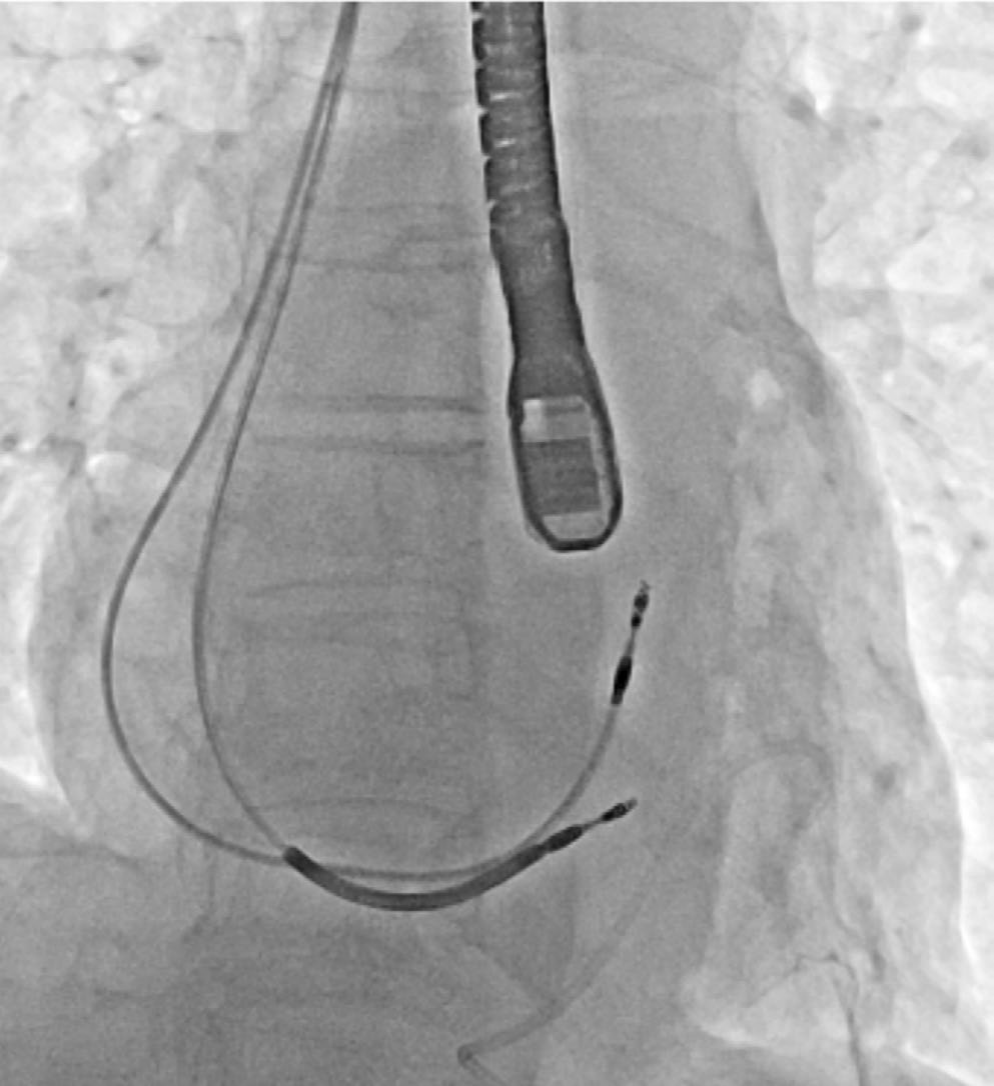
Chest radiograph after slitting the guiding catheter showing that the atrial lead was fixed adequately.
